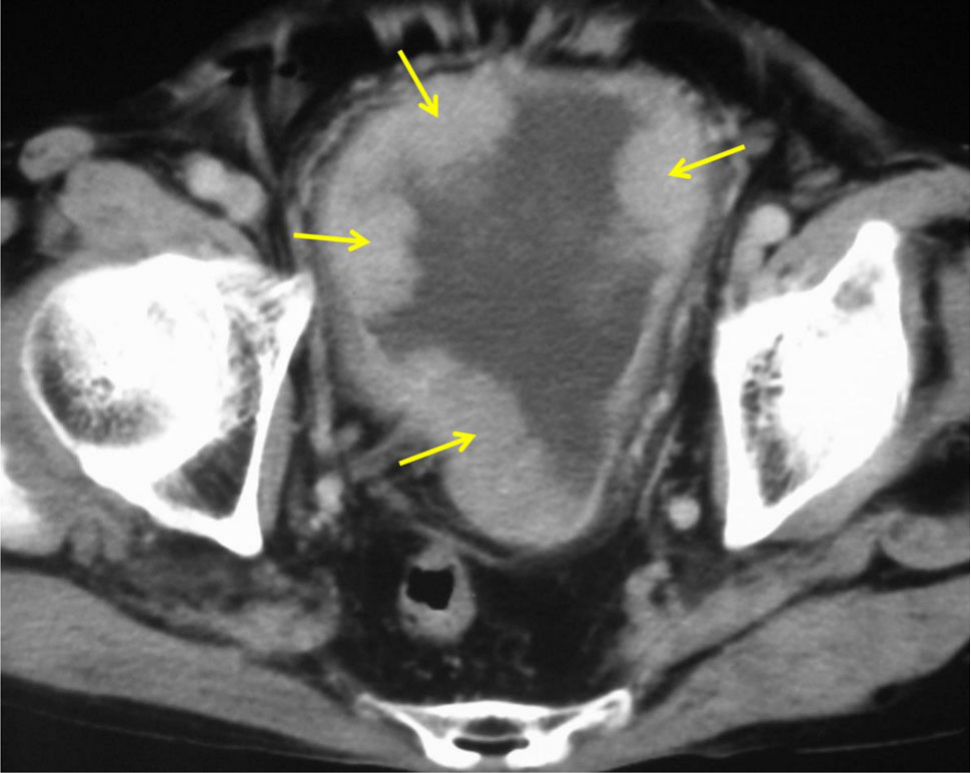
Thoracoabdominal contrast‐enhanced computed tomography. There were multiple large tumors in the bladder (arrows). There were no findings of lymph node enlargement or distant metastasis.
